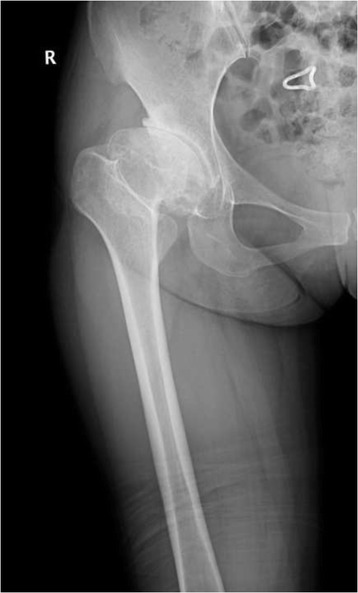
Pre-operative radiographic aspect in an anteroposterior view of a 32-year-old female with Crowe grade II developmental hip dysplasia on her right side. The patient presented with a narrow femoral canal and excessive anteversion of the femoral neck
